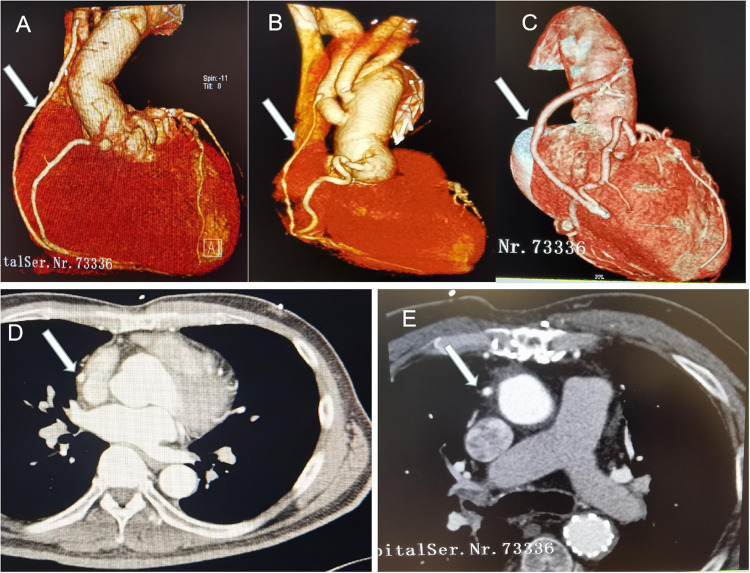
CT pictures in the follow-up time. Arrows indicate the SV bypass. (A) SV to RCA bypass+ hemi-arch replacement; (B) SV to PDA bypass + total arch replacement, SV bypass with obvious stenosis; (C) SV to right acute marginal artery bypass +hemi-arch replacement; (C,D) CT images of the SV bypass.
